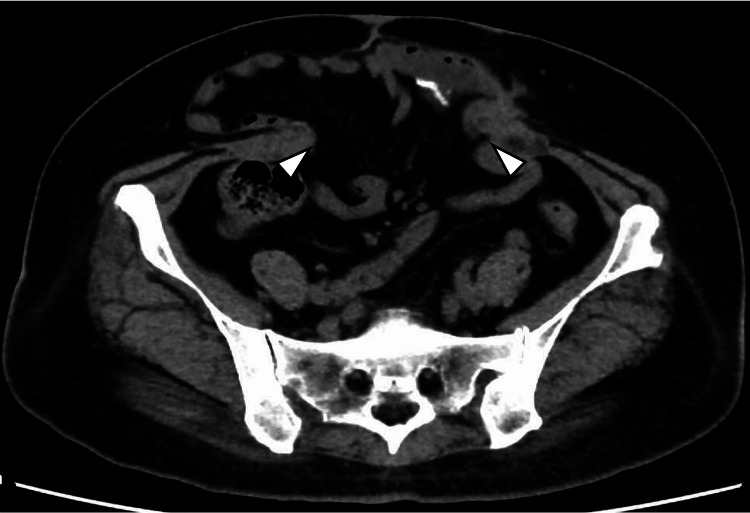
Abdominal midline incision hernia after the first surgery Contrast-enhanced computed tomography scans before the second surgery showed the existing abdominal midline incision hernia with an orifice of 18x11 cm, without recurrence of the parastomal hernia (arrowheads).
